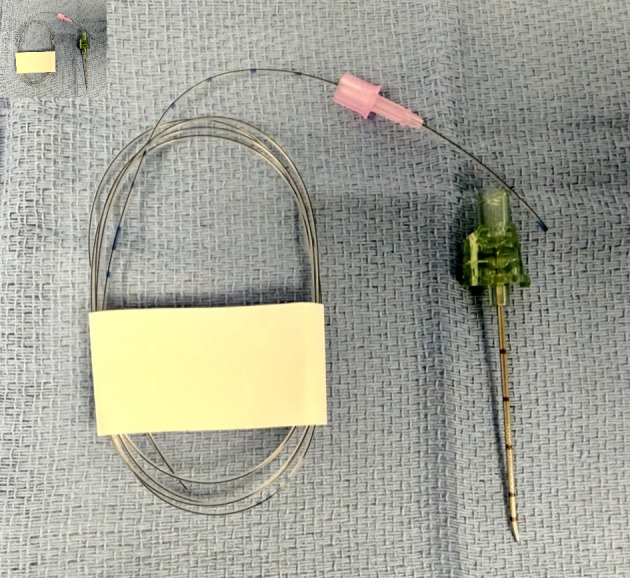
A peripheral nerve catheter from a B. Braun Contiplex^®^ Tuohy Ultra 360 set was placed under ultrasound guidance between the superior and middle trunks of the brachial plexus using an interscalene approach. The catheter was placed through a 5-cm, 18-gauge Tuohy regional blockade needle.
